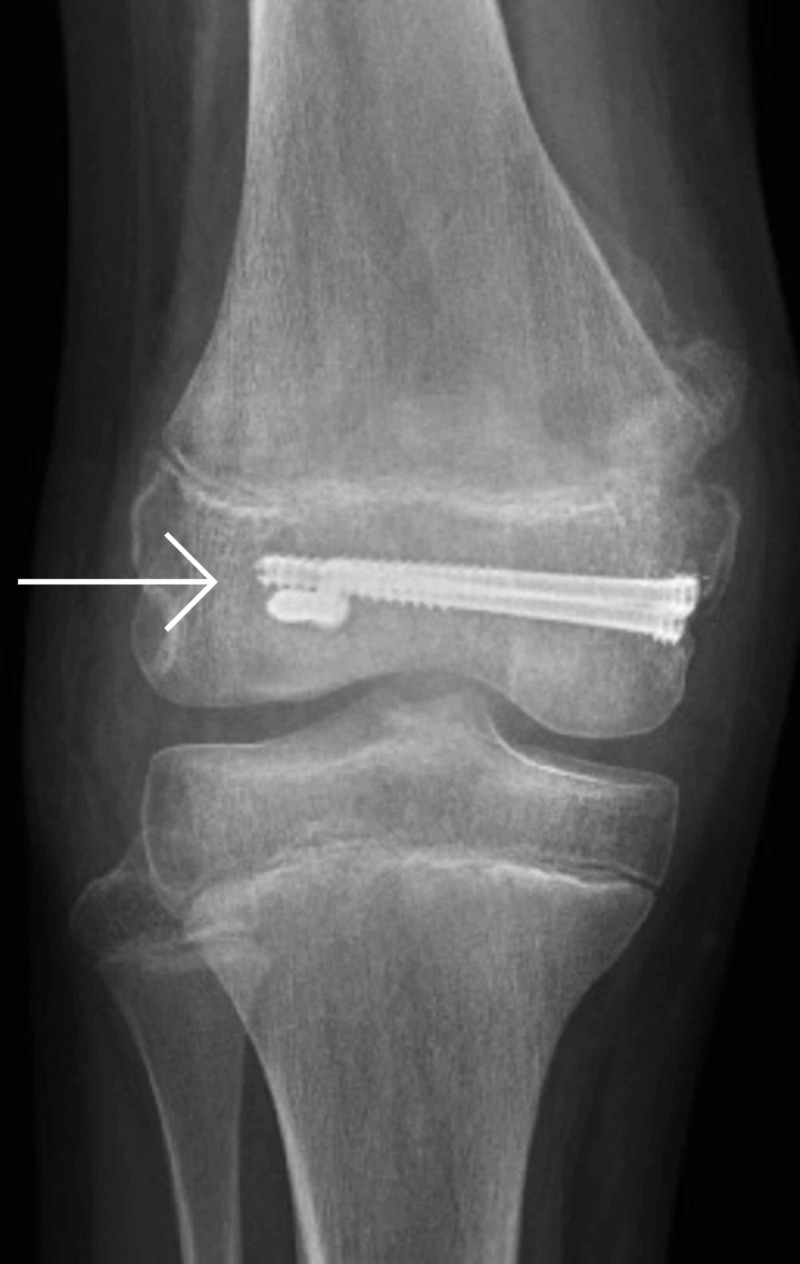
Post-operative anteroposterior x-ray post Kirschner wire removal
